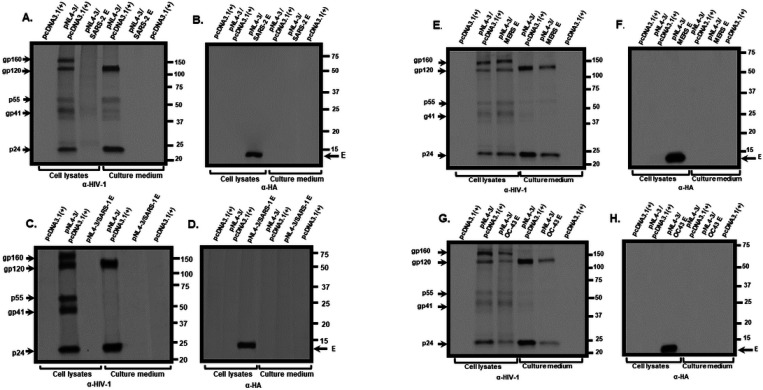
The biosynthesis of HIV-1 proteins is attenuated in the presence of β-coronavirus E proteins. HEK293 cells were transfected with either the empty pcDNA3.1(+) or vectors expressing SARS-CoV-2, SARS-CoV, MERS-CoV, or HCoV-OC43 E proteins and pNL4–3. At 30 h post-transfection, cells were starved of methionine/cysteine for 2 h and radiolabeled with ^35^S-methionine/cysteine for 16 h. The culture medium was collected, and cell lysates were prepared as described in the Materials and Methods section. HIV-1 proteins were immunoprecipitated using anti-HIV-1 antibodies while the E proteins were immunoprecipitated with antibodies directed against the HA-tag. The immunoprecipitates were collected on protein-A-Sepharose, washed, and boiled in sample-reducing buffer. The proteins were separated by SDS-PAGE and visualized using standard radiographic techniques. Panel A-B. Immunoprecipitation of HIV-1 proteins from cell lysates (A) and culture medium (B) from HEK293 cells co-transfected with either pcDNA3.1(+) alone, pcDNA3.1(+)/pNL4–3, or pcDNA3.1(+) expressing SARS-CoV-2 E-HA protein/pNL4–3. Panel C-D. Immunoprecipitation of E proteins from cell lysates (C) and culture medium (D) from HEK293 cells co-transfected with pcDNA3.1(+) alone, or pcDNA3.1(+)/pNL4–3, or pcDNA3.1(+) expressing the SARS-CoV E-HA/pNL4–3. Panel E-F. Immunoprecipitation of HIV-1 proteins from cell lysates and culture medium from HEK293 cells co-transfected pcDNA3.1(+) alone, pcDNA3.1(+)/pNL4–3, or pcDNA3.1(+) expressing the MERS-CoV E-HA/pNL4–3. Panel G-H. Immunoprecipitation of HIV-1 proteins from cell lysates and culture medium from HEK2933 cells co-transfected pcDNA3.1(+) alone, pcDNA3.1(+)/pNL4–3, or pcDNA3.1(+) expressing the HCoV-OC43 E-HA/pNL4–3.
